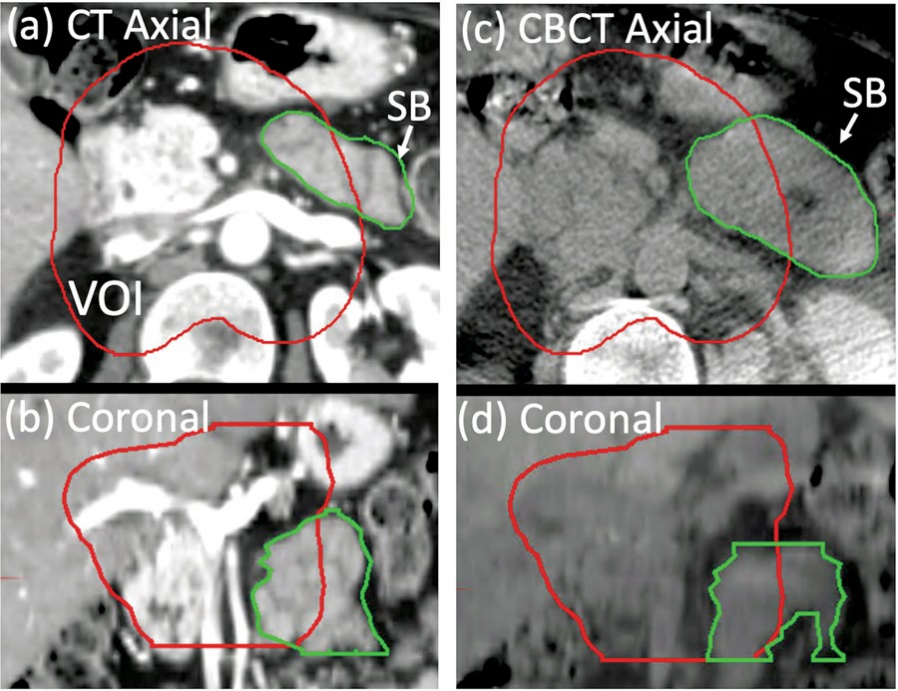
Example of physician-drawn small bowel (SB) contours on (a) axial and (b) coronal views of the planning CT, and on corresponding views (c), (d) of the CBCT scan. The volume of interest (VOI) is also outlined.
